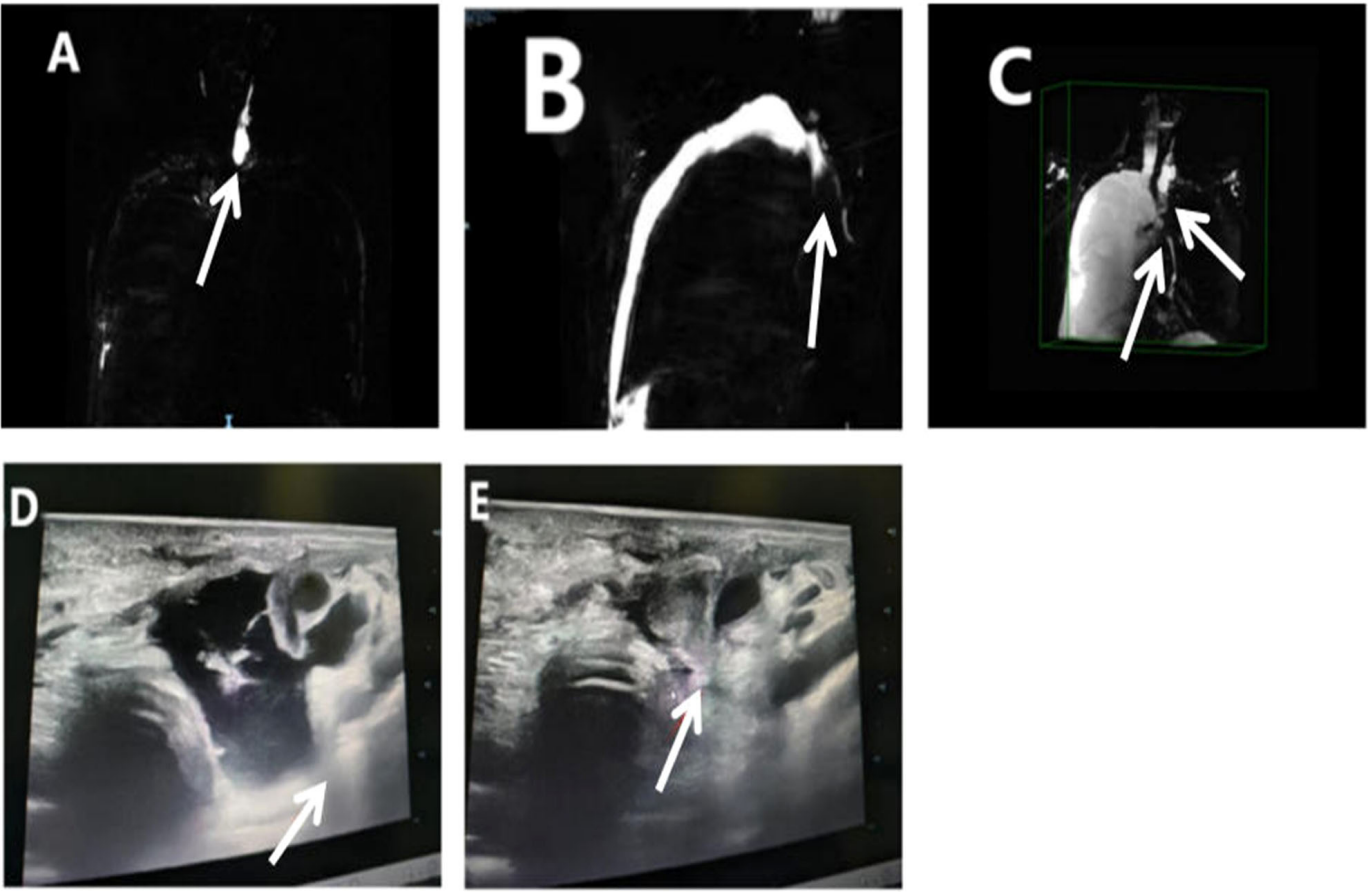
Lymphangiography, MRI, and ultrasound-guided intervention. (A) T2-weighted MR lymphangiography showing fluid accumulation in the left thyroid bed with communication to the left cervical lymphatic trunk (white arrow). (B, C) Coronal and 3D reconstructed images demonstrating communication between a right thoracic duct branch and the right pleural effusion (white arrows). (D) Pre-puncture ultrasound image revealing cervical lymphatic fluid collection and a suspected leak channel (white arrow). (E) Under real-time ultrasound guidance, 76% meglumine diatrizoate was locally injected at the leak site (needle tip indicated by the white arrow).
